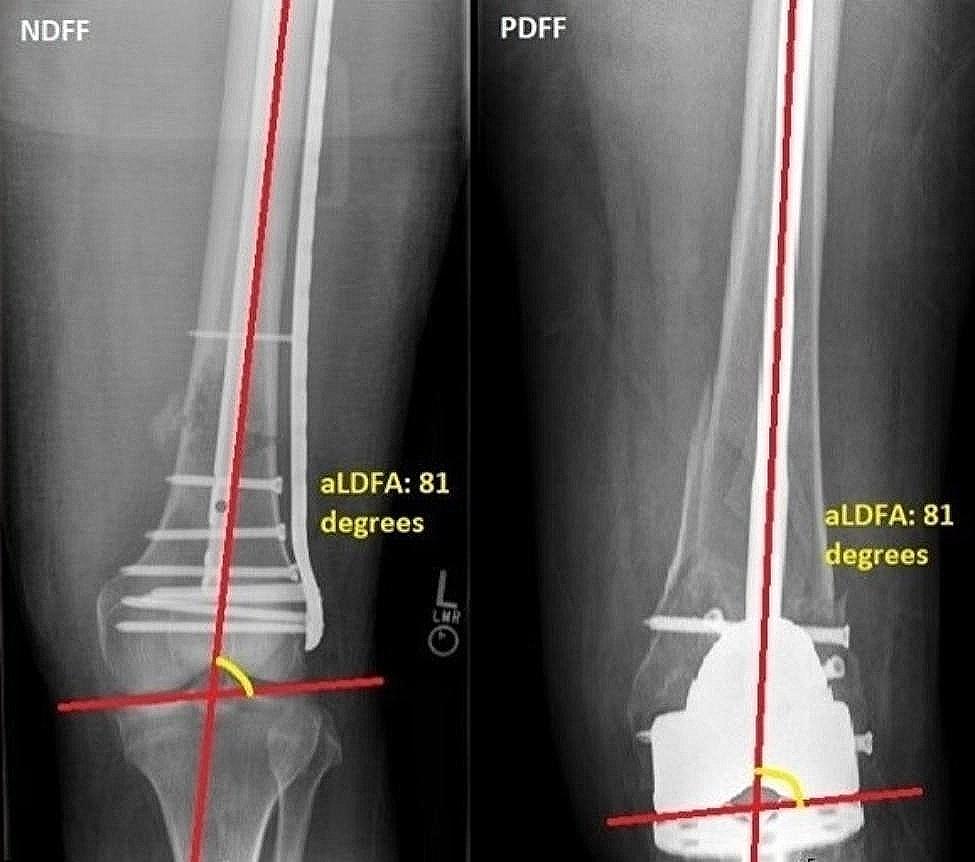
Measurement of aLDFA on AP radiographs
